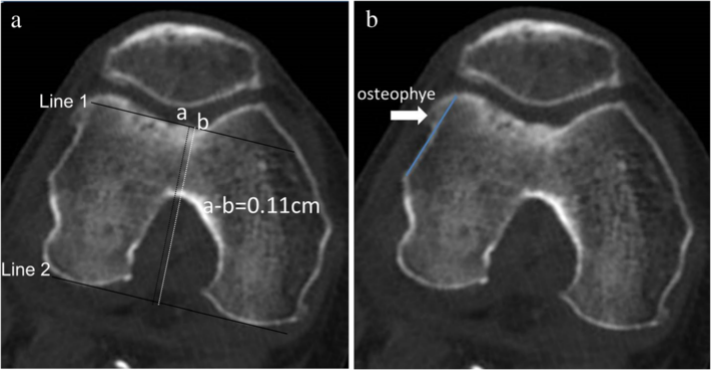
Preoperative computed tomography (CT) scanning. a the measurement of distances from the lowest point of trochlear groove to the midpoint of anterior condyle in CT images. Line 1: the virtual anterior condyle osteotomy line; line 2: the posterior condyle line. b the osteophytes in the patients with severe knee osteoarthritis
